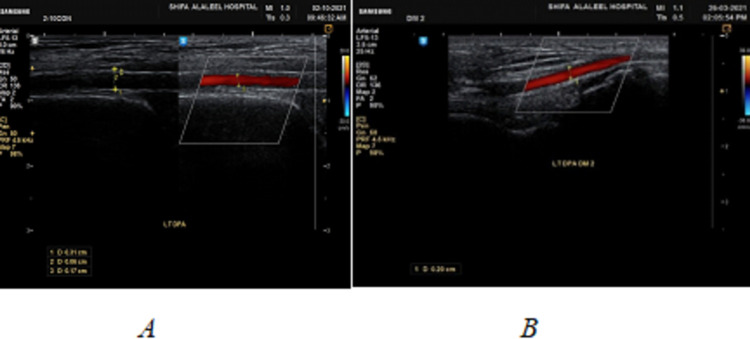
A longitudinal portion of a Doppler color sonography of the left DPA demonstrates blood flow lumen diameter measurement (A) in the control group and (B) in the diabetes group. DPA: dorsalis pedis artery
